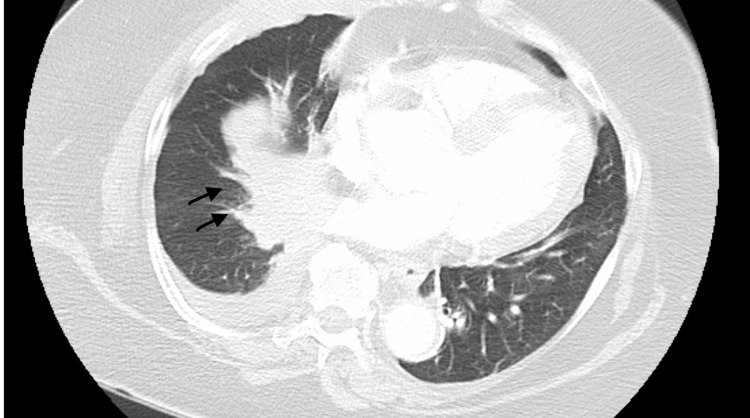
Initial CT chest with contrast showed hilar involvement of mass.
